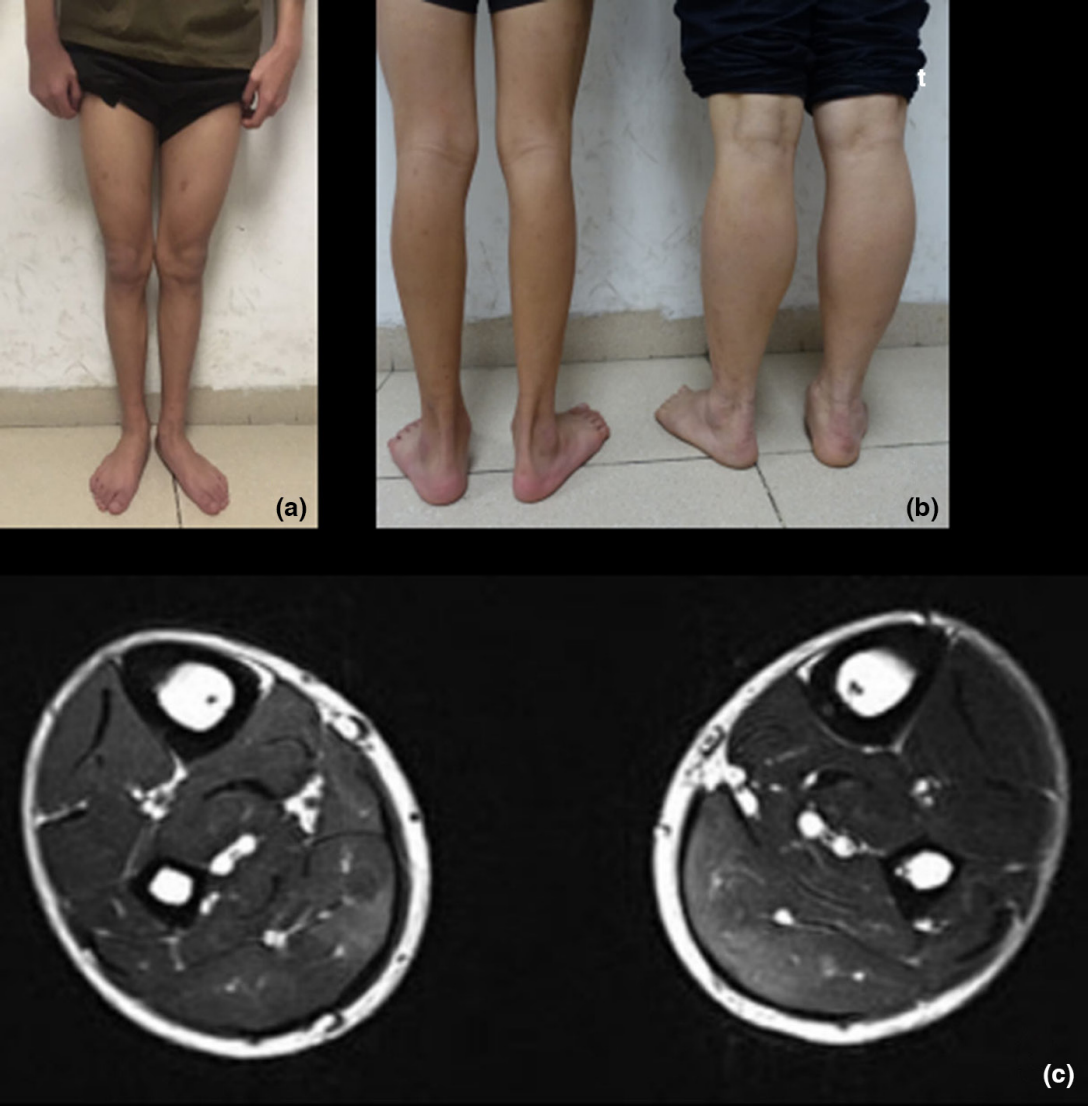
(a) Physical examination showed no atrophy of muscle, but the lower limbs of the patient (left) were thin, (b) comparison of the lower limbs of the father (right), (c) lower‐limb muscle magnetic resonance imaging showed increased signal for the bilateral soleus and musculi flexor pollicis longus on T2‐weighted images.
